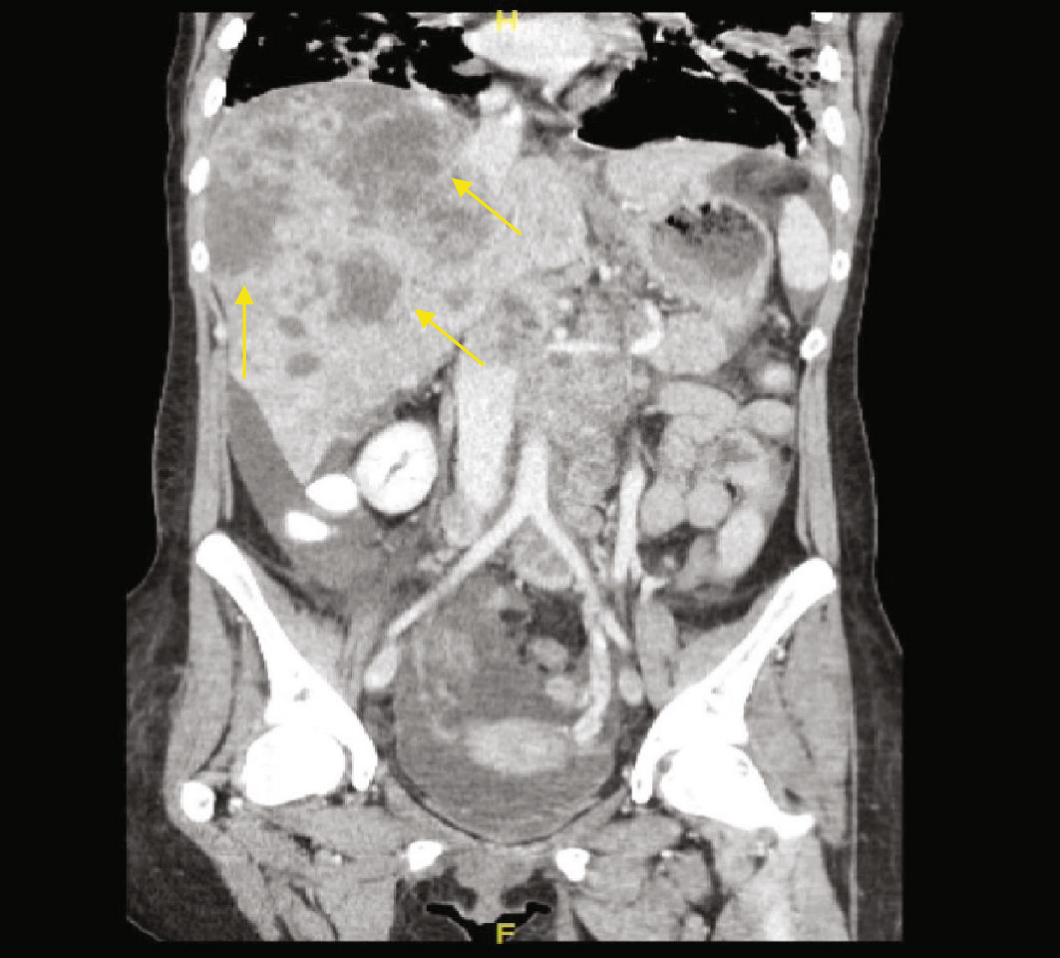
Computed tomography scan showing liver metastasis along with retroperitoneal, mesenteric, and celiac lymphadenopathy.
